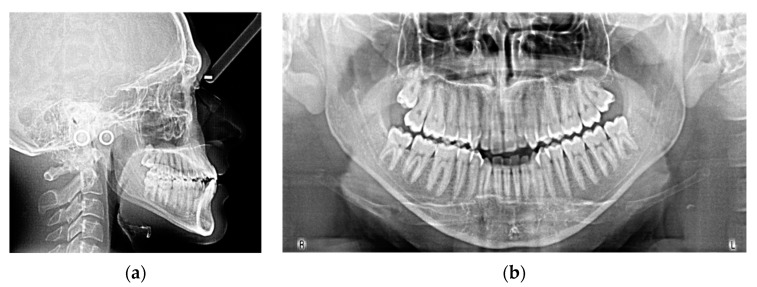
Lateral cephalogram (a) and panoramic radiograph (b) at the beginning of the treatment.
